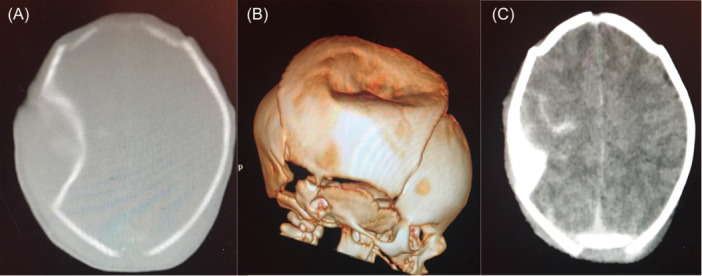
Preoperative (A) axial Computed tomography (CT) scan bone window, (B) 3D CT scan, and (C) plain CT scan. Images show a large frontoparietal ping pong fracture of 7 × 5 × 2 cm in diameter, compressing the underlying brain structure and associated with traumatic subarachnoid hemorrhage.
